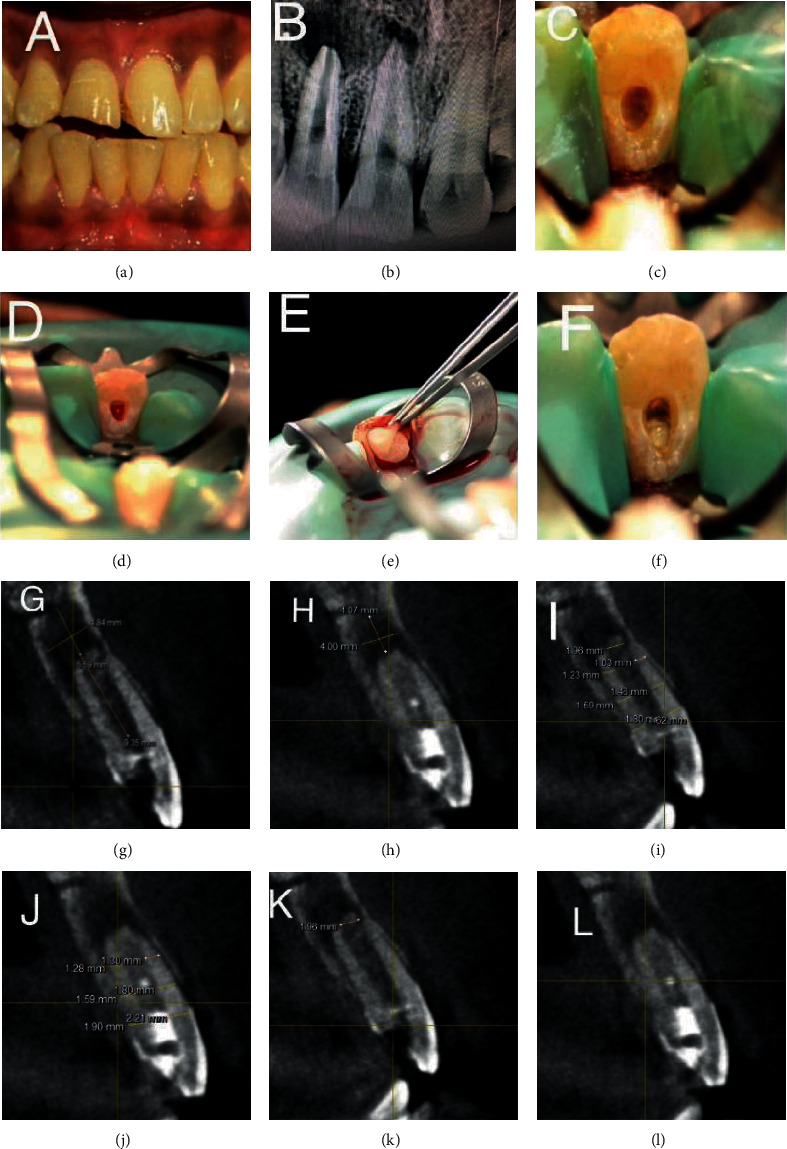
Preoperative clinical, revascularization procedure and radiographic images—IOPA and CBCT images (case report 1). (a) Pre-op clinical photograph showing fractured of 11 and 12. (b) Preoperative IOPA showing the periapical lesion involving the apices of 11 and 12 and immature roots of 12. (c) Access opening done in 12. (d) Bleeding induced in 12 for revascularization. (e) Placement of CGF in the root canal space. (f) MTA plug of 3 mm placed at the level of CEJ in 12. (g) Pre-op CBCT image showing the extent of lesion in sagittal slice. (h) Post-op CBCT image showing the extent of lesion in sagittal slice. (i) Pre-op CBCT showing the root dentin thickness measurement in sagittal slice. (j) Post-op CBCT showing the root dentin thickness measurement in sagittal slice. (k) Pre-op CBCT showing the apical diameter measurement in 12. (l) Post-op CBCT showing the apical diameter in 12.
